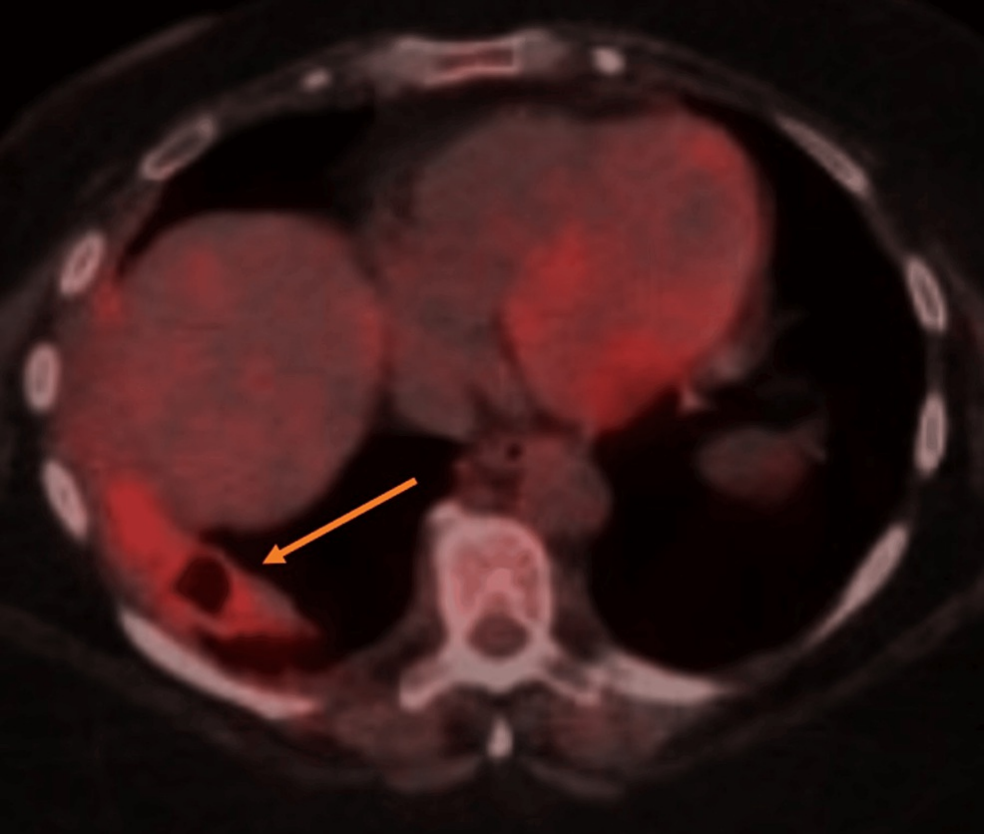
Follow-up PET/CT Cavity decreased in size; radiotracer uptake was noted. Cross-section includes liver. PET, positron emission tomography
